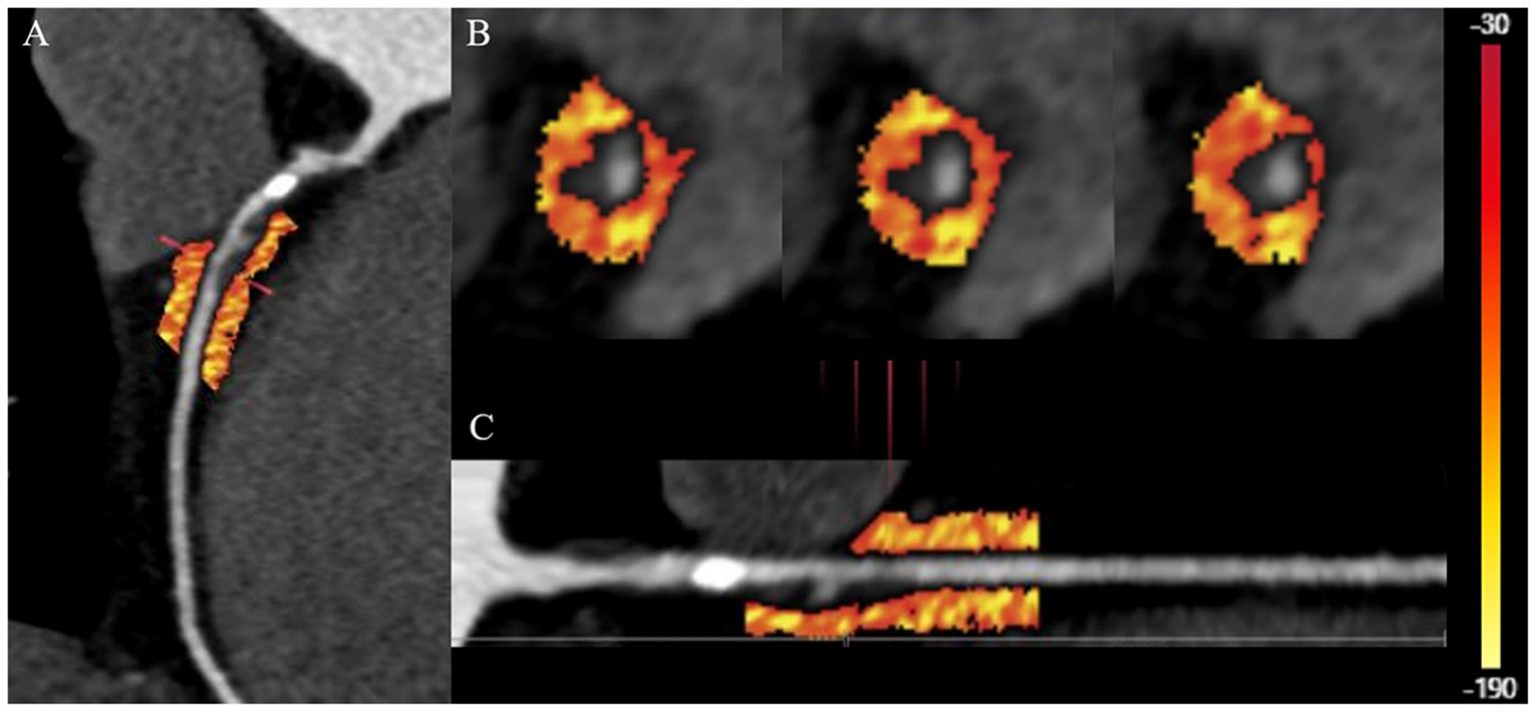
Quantification of PCAT CT attenuation of low-attenuation plaques in the proximal-mid right coronary artery. (A) The multiplanar reconstructed image of PCAT measured with the range from −190 to −30 HU. (B) Cross-section images of PCAT measure. (C) Straightened image of PCAT measure. PCAT, pericoronary adipose tissue; HU, Hounsfield units.
